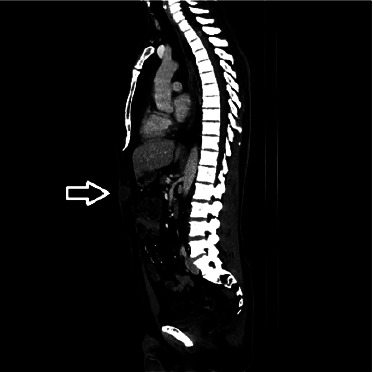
Sagittal computed tomography—tumor in the abdominal wall.
